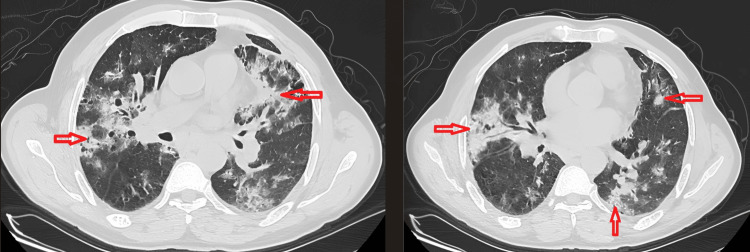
Chest CT scan Chest CT scan showing bilateral ground-glass opacities and alveolar consolidations with basal and middle lobe predominance, in the parenchymal window. CT, computed tomography
